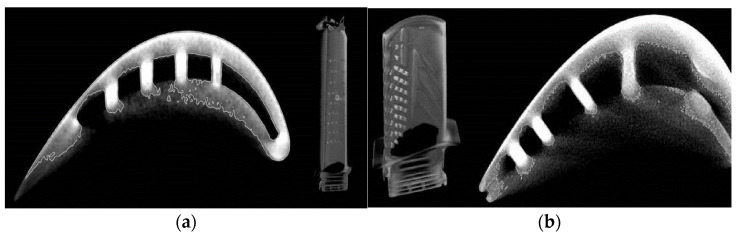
The shape of the LPT blade (a) and the HPT blade (b), with visible cooling channels, obtained by the computer-based micro-tomography CT.
